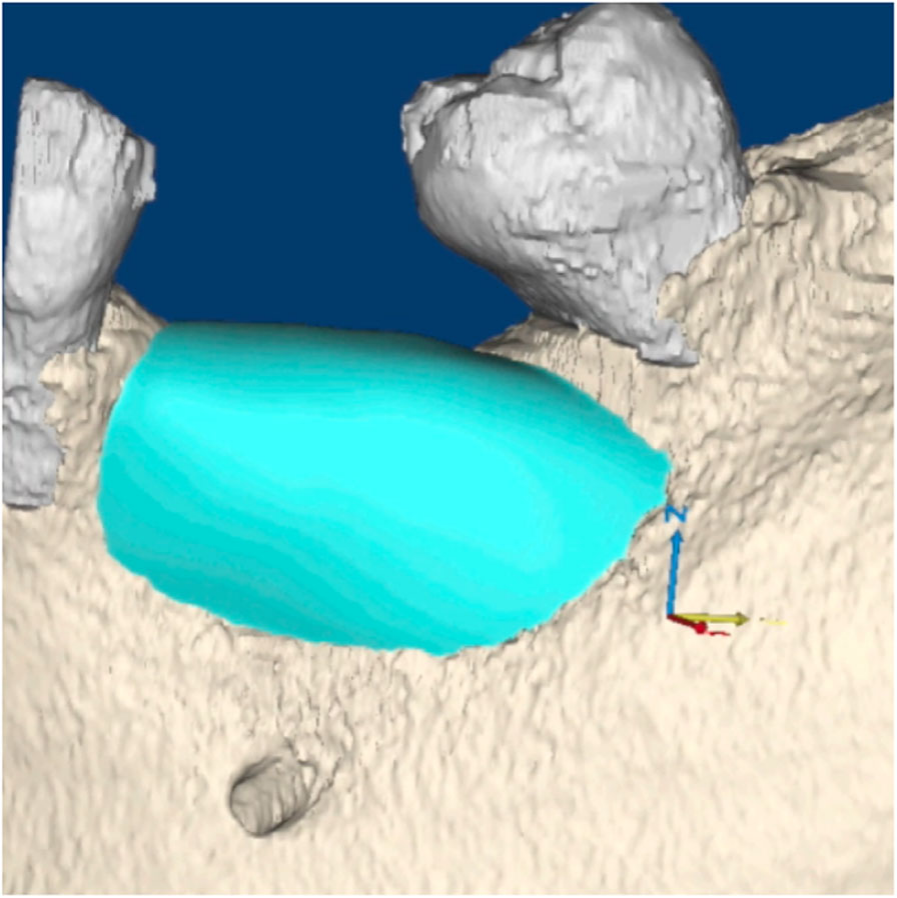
“Ideal” osseous contour digitally added (blue) to the 3D reconstruction of the CBCT scan
